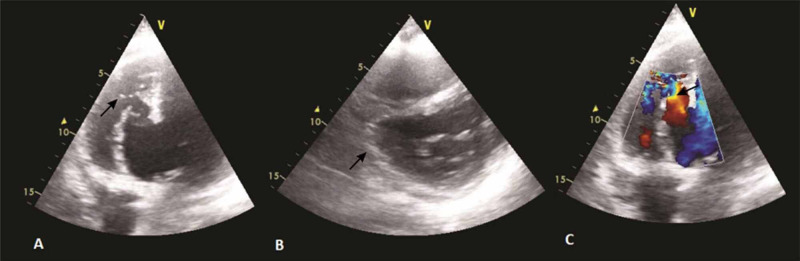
Echocardiography images Four-chamber view showing the aneurysm with ventricular septal rupture (VSR) (A); parasternal short-axis (PSAX) view showing aneurysm at right coronary artery (RCA) (B); Colour doppler showing flow across VSR (C)
